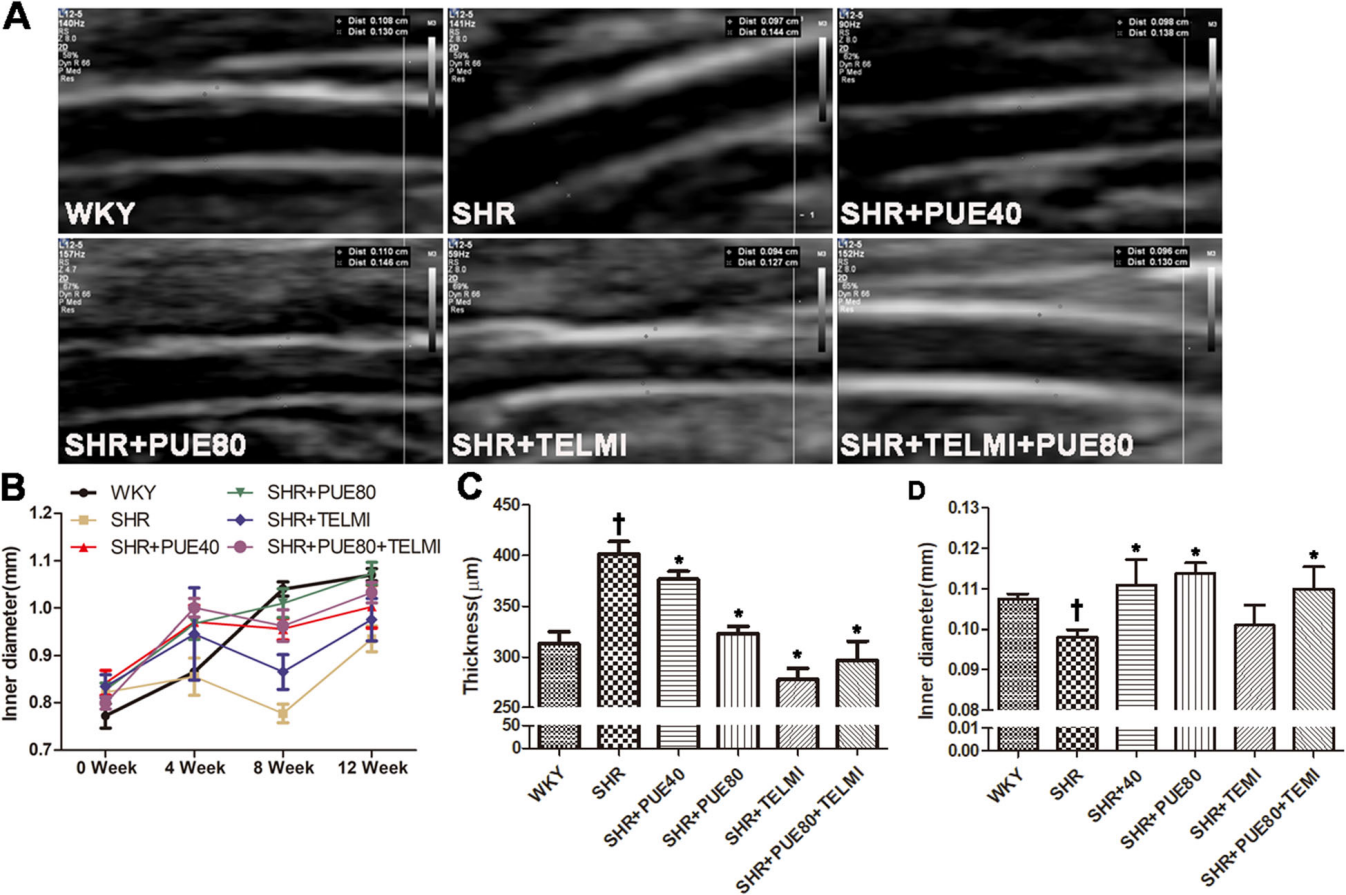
Effect of PUE in common carotid artery with ultrasound image. A A representative ultrasound record of CCA at the end of 12 weeks. B Inner diameter changes of CCA with PUE during 0–12 weeks. C Histogram analysis of thickness changes of CCA with PUE at the end of 12 weeks. D Histogram analysis of inner diameter changes of CCA with PUE at the end of 12 weeks. † *p* < 0.05 vs WKY, * *p* < 0.05 vs SHR. (Repeated measures ANOVA)
